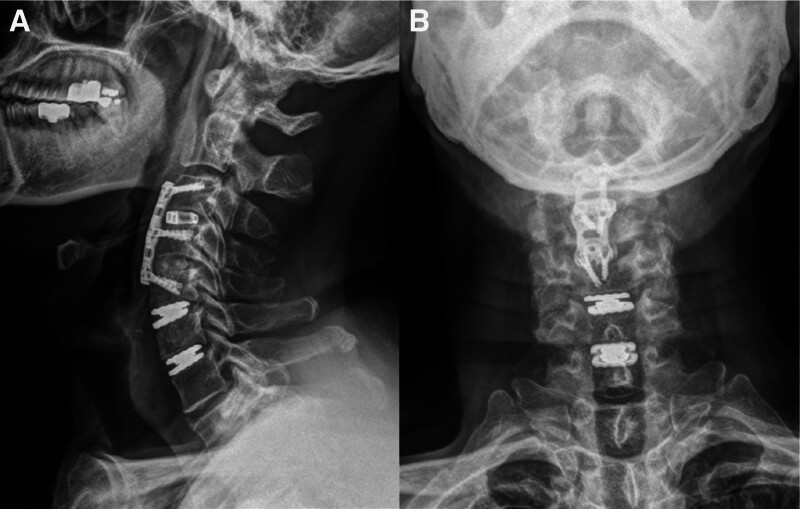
Postoperative cervical spine X-ray image. (A) Lateral and (B) Anterior–posterior projection showing anterior cervical fusion at the C3–C5 level and artificial disc replacement at the C5–C7 level.
